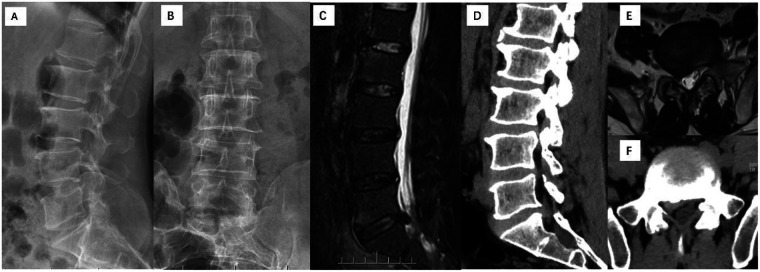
Preoperative imaging findings of the patient. (A,B) Preoperative anteroposterior and lateral radiographs. (C) Preoperative sagittal T2-weighted MRI. (D) Preoperative sagittal CT. (E) Preoperative axial T2-weighted MRI. (F) Preoperative axial CT.
